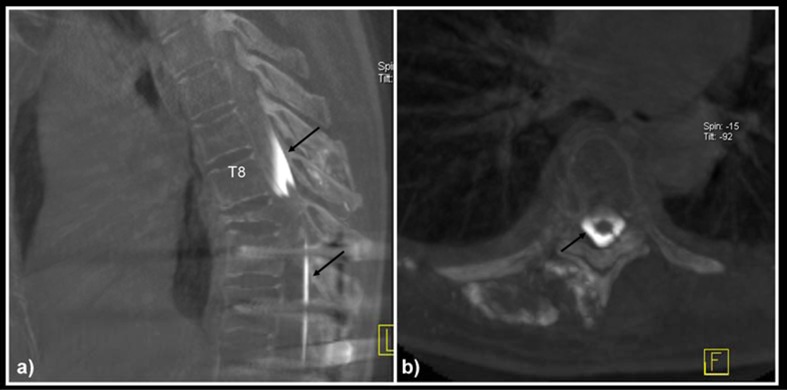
Dyna-CT on: a) sagittal and b) axial view confirmed tumoural bleed which extends into the epidural and intrathecal space (arrows).
